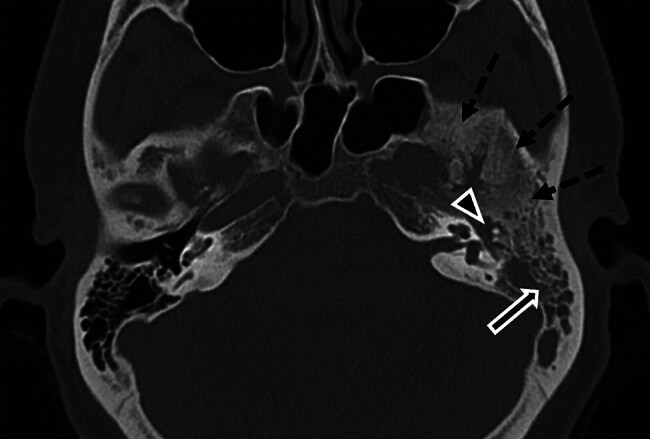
Axial computed tomography (CT) image through the bilateral temporal bones demonstrates trapped fluid within the left mastoid air cells (solid arrow), soft tissue attenuation within the middle ear cavity (arrowhead), and diffuse sclerosis and hyperostosis of the left lateral and middle skull base (dashed arrows). Note the normal right side.
